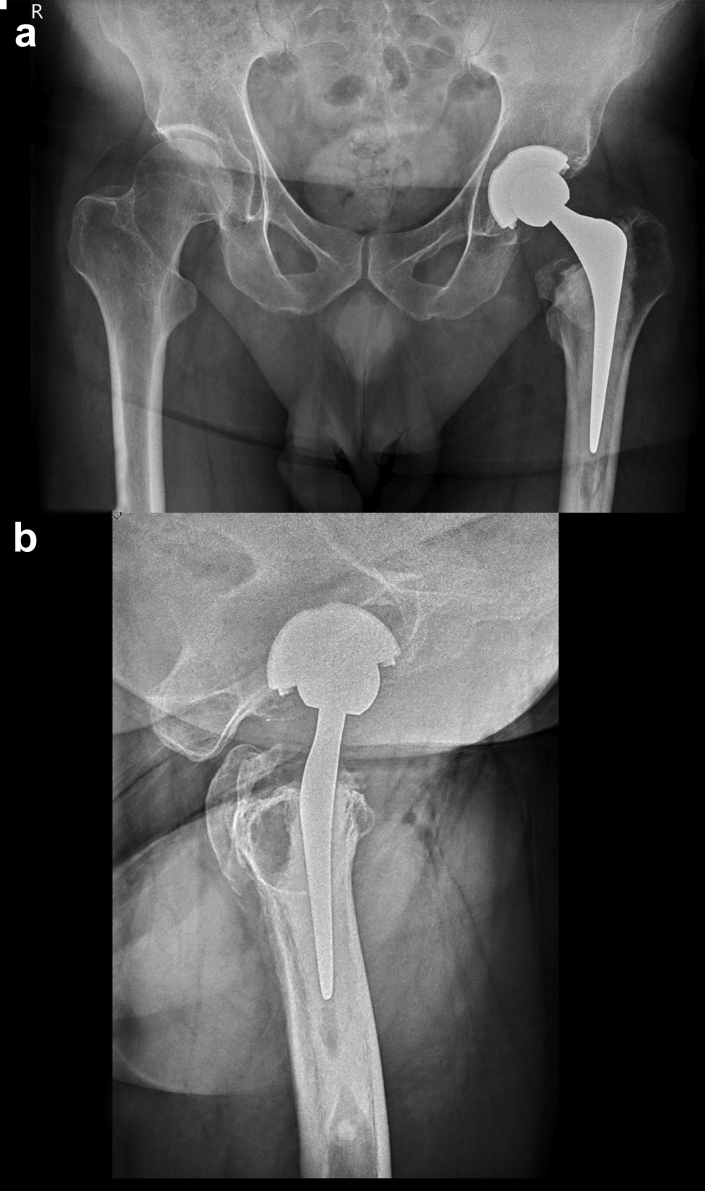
Plain anteroposterior (a) pelvic and lateral (b) hip radiographs after the revision surgery. Note the smaller implant compared to figure 3.
